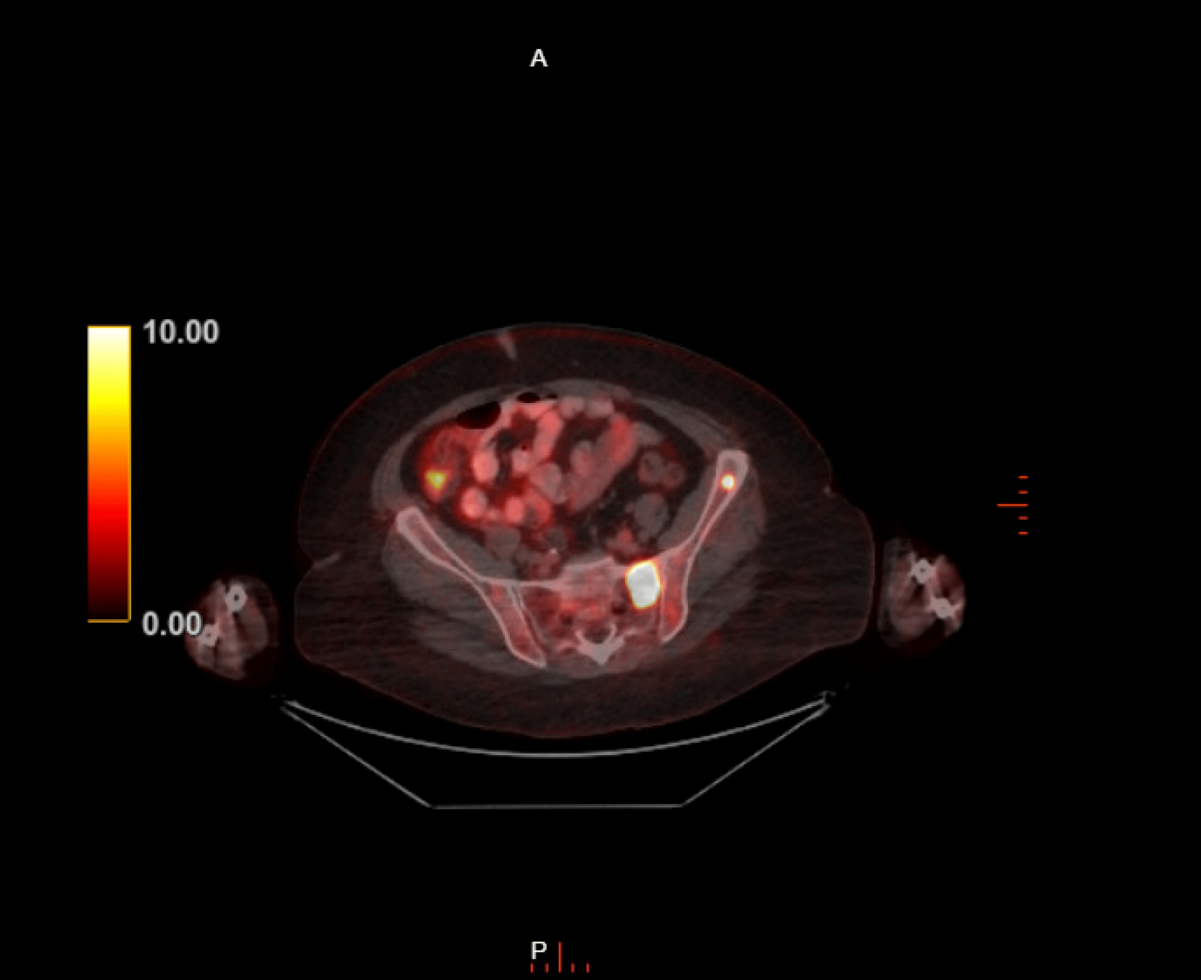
Positron emission tomography CT scan with focally FDG-avid lesion within the left iliac wing FDG: fluorodeoxyglucose, CT: computed tomography
